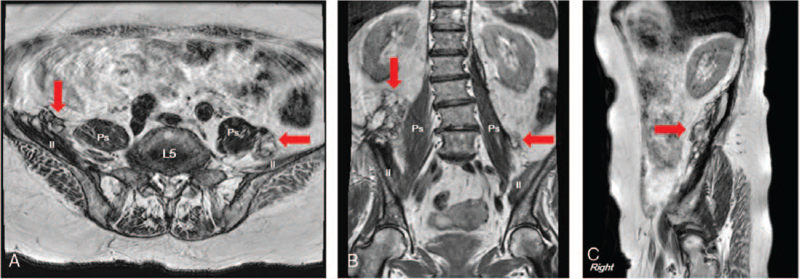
Pelvic magnetic resonance imaging at follow-up. (A) Axial plane at the lumbar vertebra 5 level; (B) coronal plane; (C) sagittal plane. The red arrows indicate a decrease in the extent of the previously noted liquefied hematoma in the right retroperitoneum and mostly resorbed hematoma in the left retroperitoneum. Il = iliacus muscle, L5 = 5th lumbar vertebra, Ps = psoas muscle.
